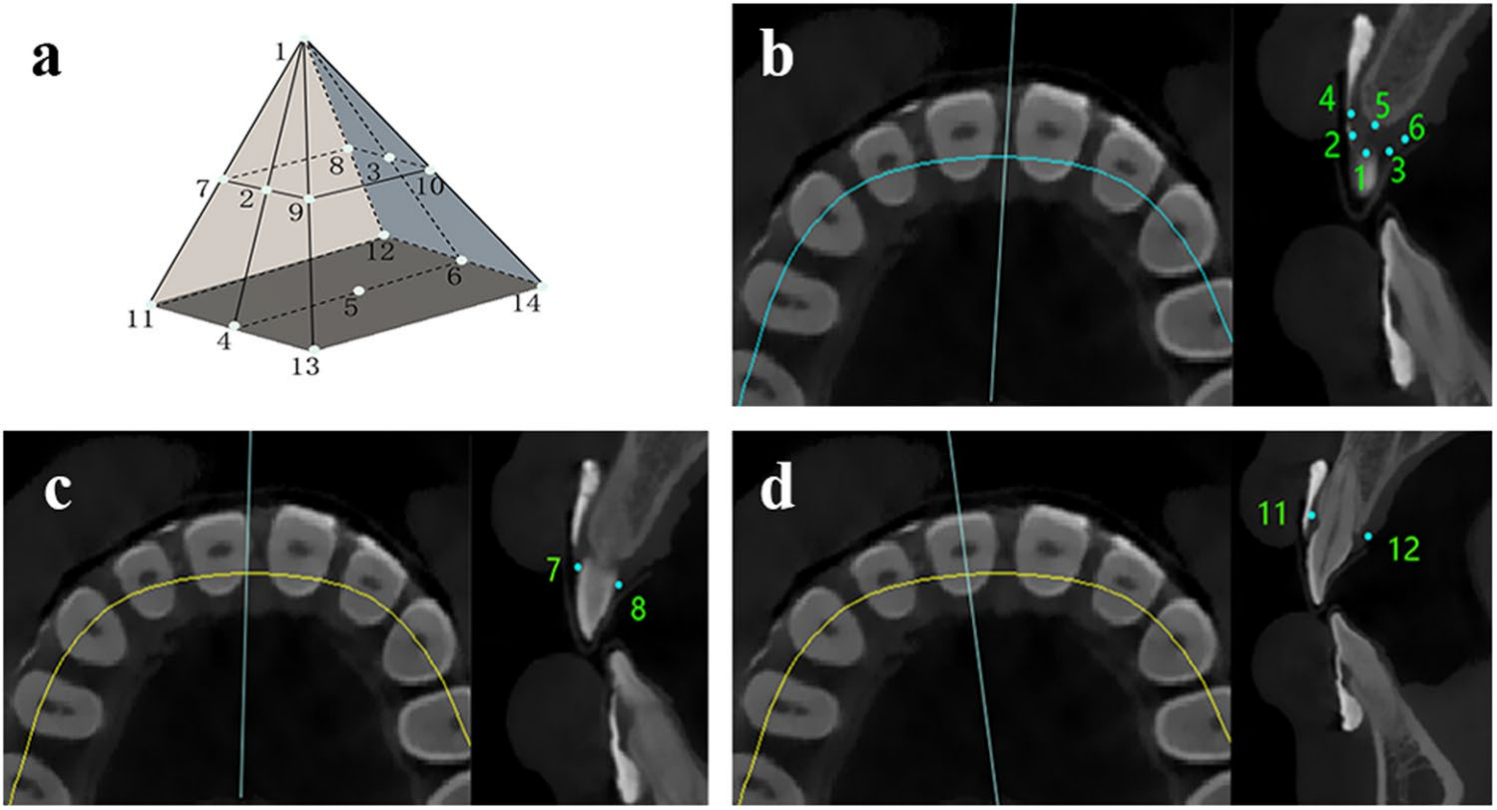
3D imaging reconstruction and the measurements of PV. After CBCT scanning and 3D imaging reconstruction, interdental papilla was defined as a quadrangular pyramid outlined by 14 points (a). Points 1–6 were located in the mid-cross-sectional plane perpendicular to the connecting line of two neighbouring teeth (b). Points 7–10 were located at the free gingival margin in the proximal surfaces of the teeth (c). Points 11–14 were located in the cross-sectional image that passed through the long axis of the tooth and was perpendicular to labial surfaces (d).
